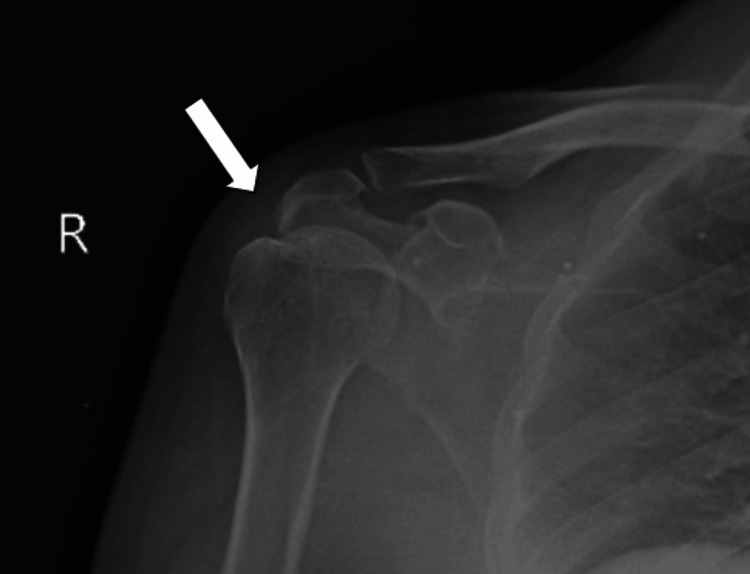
X-ray of the right shoulder joint signifies supraspinatus partial tear The arrow represents supraspinatus muscle partial tear
